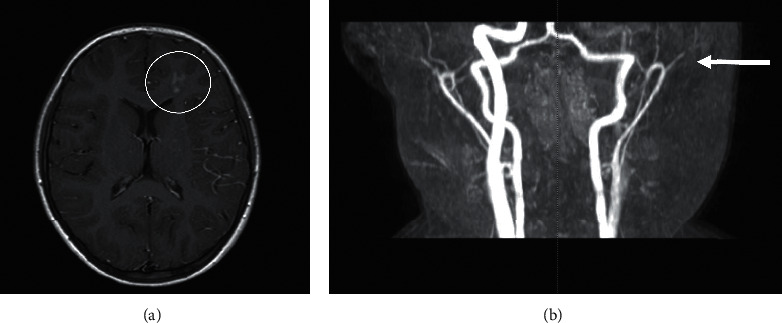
Brain neuroimaging results. (a) MRI: ischemic injury in the left frontoparietal region. (b) MRA: stenosis tightened by the left ICA and the thinning and irregularity of the left MCA.
